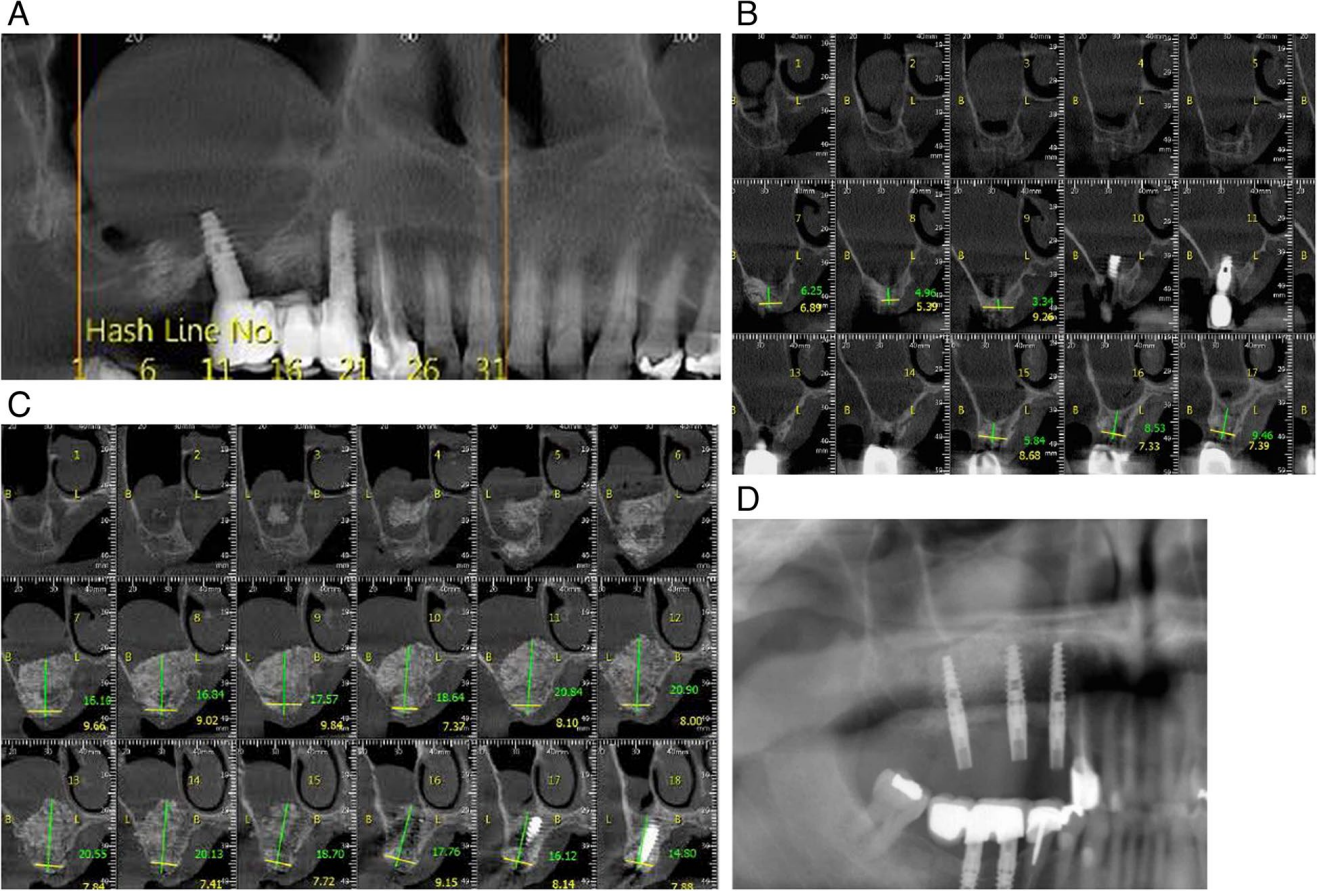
A The dental implants were positioned in the maxillary arch at position 14 and 16. The panoramic image before extraction shows polyp in the right maxillary sinus and peri-implantitis surrounding the implant. Both implants are indicated for removal due to their decreased stability and vertical and horizontal mobility at stage 3. B Coronal CBCT section shows the implants in the maxillary sinus with a minimal alveolar bone level of 3.5–9 mm in the vertical dimension. C The coronal CBCT section after graft placement shows that new bone has formed over a period of five months. D The panoramic image shows that the three new implants are seated in the alveolar bone at positions 14, 15, and 17 and demonstrate primary stability on clinical examination
